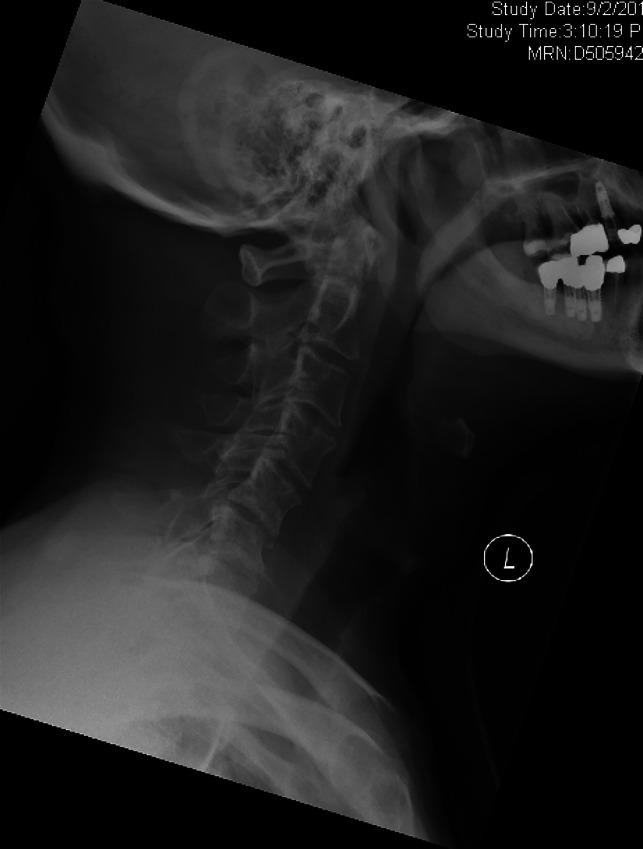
Radiograph showing instability in the HK case.
